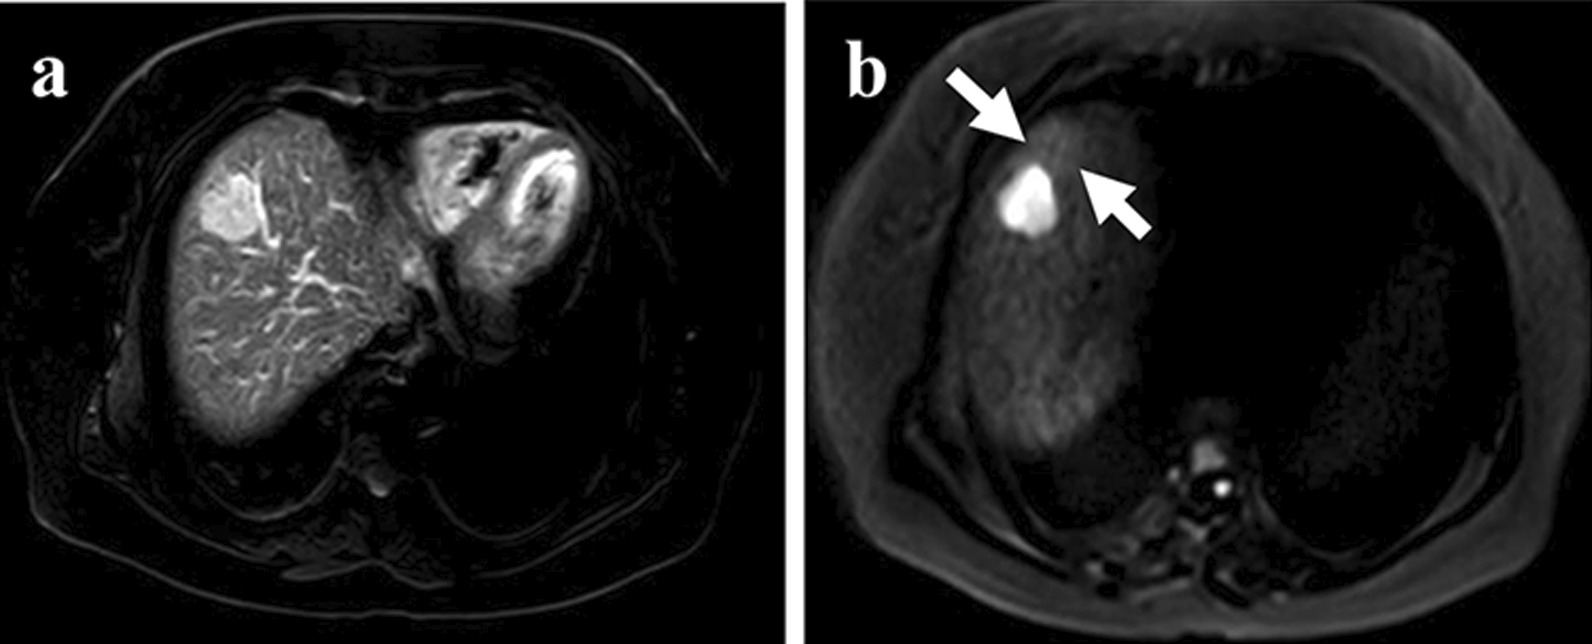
A 54-year-old woman with sHCC with MVI. a T2WI shows a high signal intensity hepatic mass with round shape and smooth margins. b DWI shows mismatch region compared to T2WI (thin arrow)
